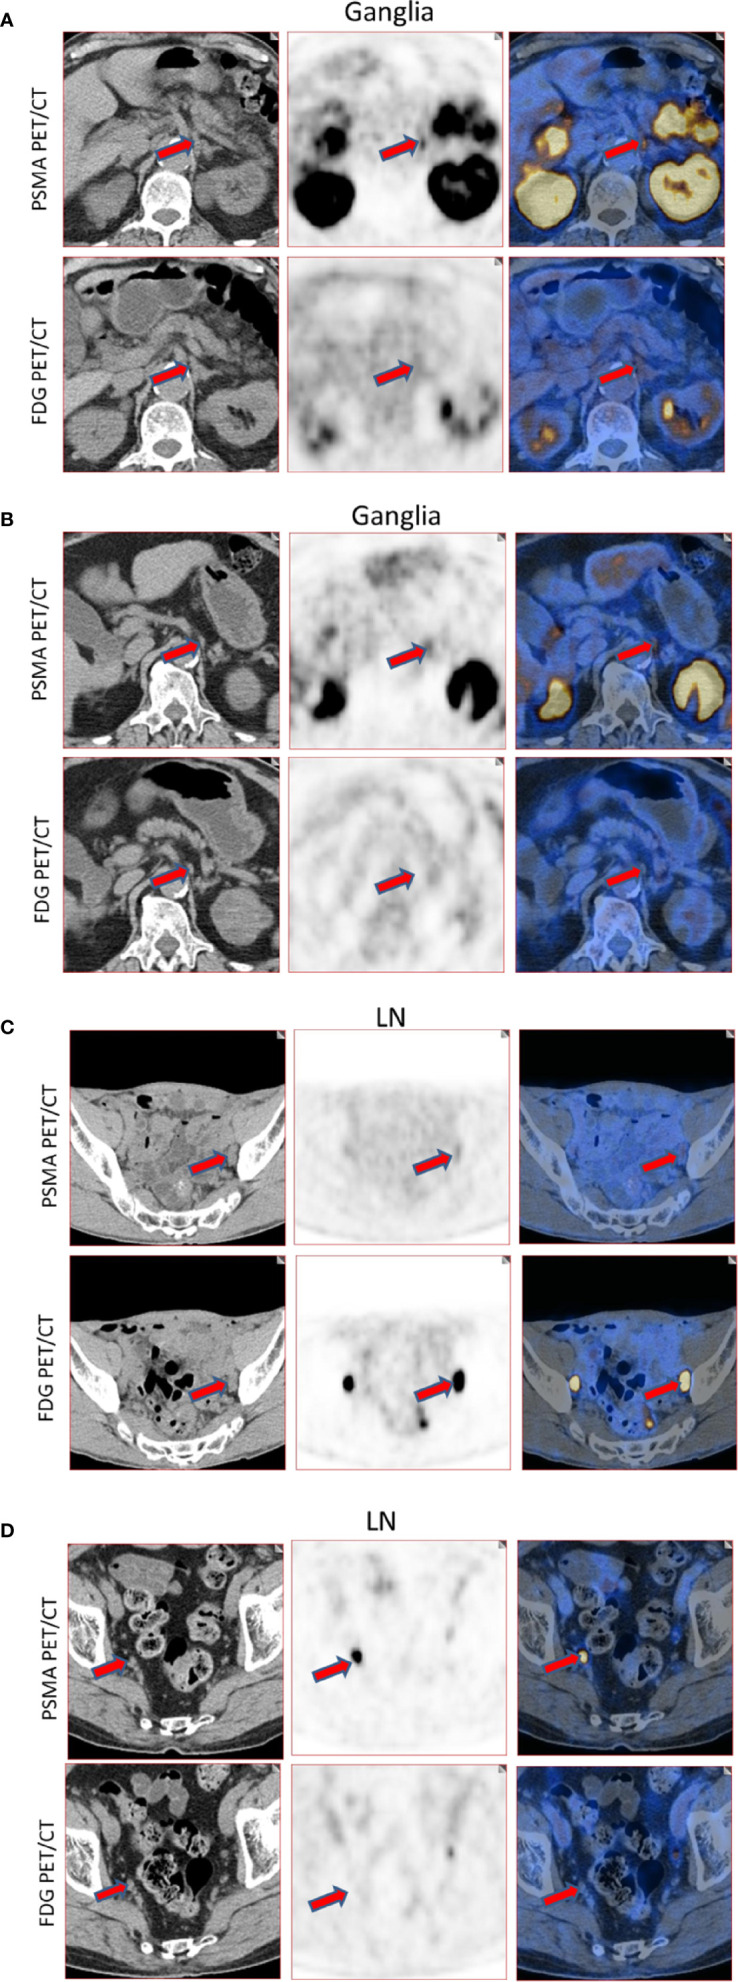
Representative images of PSMA-11-positive ganglia and lymph node metastases. (A) FDG-positive celiac ganglia (red arrow, SUVmax of PSMA-11-PET 6.6, SUVmax of FDG-PET 2.9). (B) FDG-negative celiac ganglia (red arrow, SUVmax of PSMA-11-PET 3.6, SUVmax of FDG-PET 0.7). (C) FDG-positive pelvic lymph node metastasis (red arrow, SUVmax of PSMA-11-PET 3.2, SUVmax of FDG-PET 28.0). Lymph node metastasis was confirmed by postoperative pathology. (D) FDG-negative pelvic lymph node metastasis (red arrow, SUVmax of PSMA-11-PET 14.4 and SUVmax of FDG-PET 0.6). Lymph node metastasis was confirmed by postoperative pathology.
